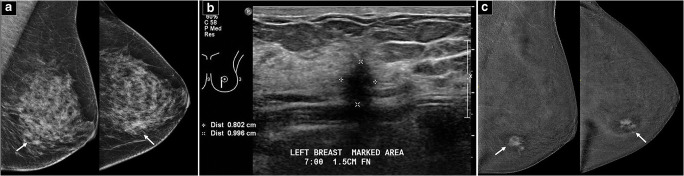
Example of group 1 classification. a Full-field digital mammograms (FFDM) of both breasts (CC and MLO views) demonstrate a mass with irregular margins in the left lower inner breast (arrows). b Ultrasound of the left breast confirms a suspicious 10-mm hypoechoic mass at the 7OC position, 1.5 cm from the nipple. G2 invasive ductal carcinoma (IDC) (ER/PR+, Her2−) was proven on biopsy. c CC and MLO views of contrast-enhanced digital mammogram (CEM) demonstrate identical findings to FFDM and ultrasound. There is a solitary 1.2-cm irregular enhancing mass in the left lower inner breast (arrows). No new findings on CEM were detected (group 1 classification). The patient underwent a wide local excision which revealed a 1.2-cm G2 IDC
